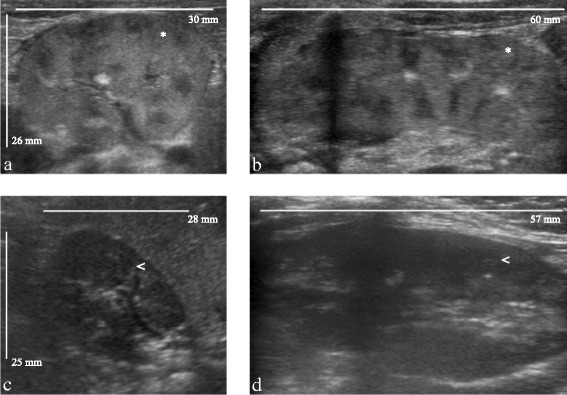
Comparison of renal ultrasounds at birth (a, b) and at the age of 8 months (c, d) in a patient with propionic acidemia. At birth, the patient presented enlarged kidneys with hyperechogenic cortexes (*). (a: transverse plane, b: longitudinal plane). At the age of 8 months, kidney size was normal, and the cortexes were normoechogenic (<). (c: transverse plane, d: longitudinal plane)
